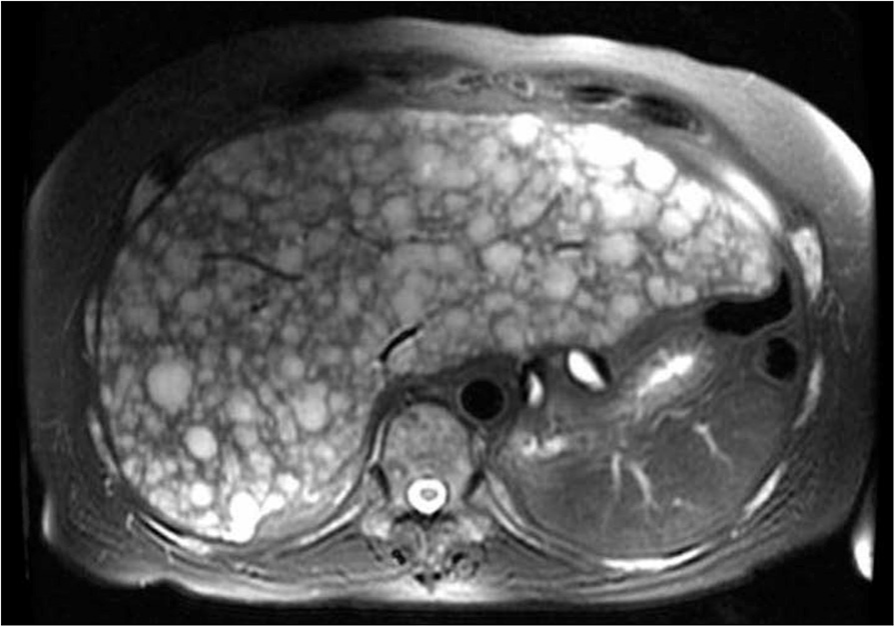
MRI Liver at the time of clinical progression. Axial T2-weighted MRI shows interval development of innumerable solid lesions in the liver, replacing a majority of the parenchyma in both hepatic lobes with development of a pseudocirrhotic appearance of the liver with a nodular surface contour
